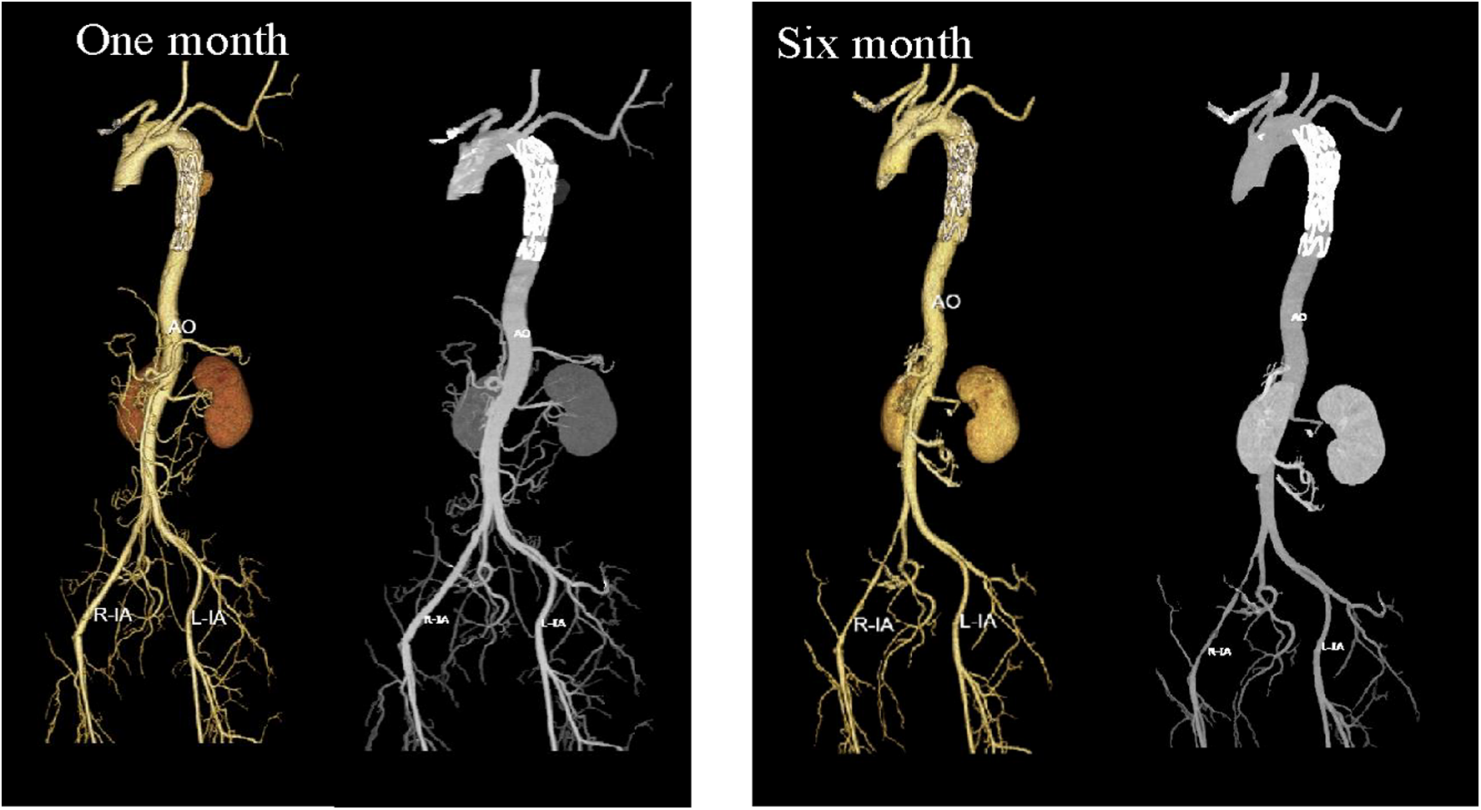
Three-dimensional reconstruction images of postoperative CTA at one month and six months suggested good stent positioning, with no visible evidence of the primary lesion.
